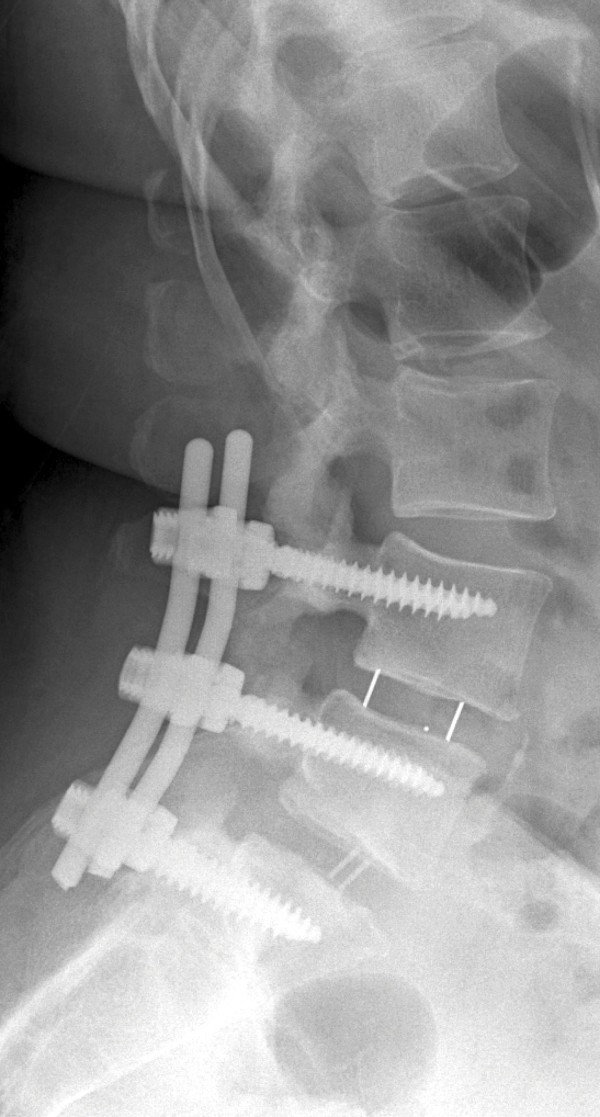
X-ray taken at the patient’s second follow-up examination.
